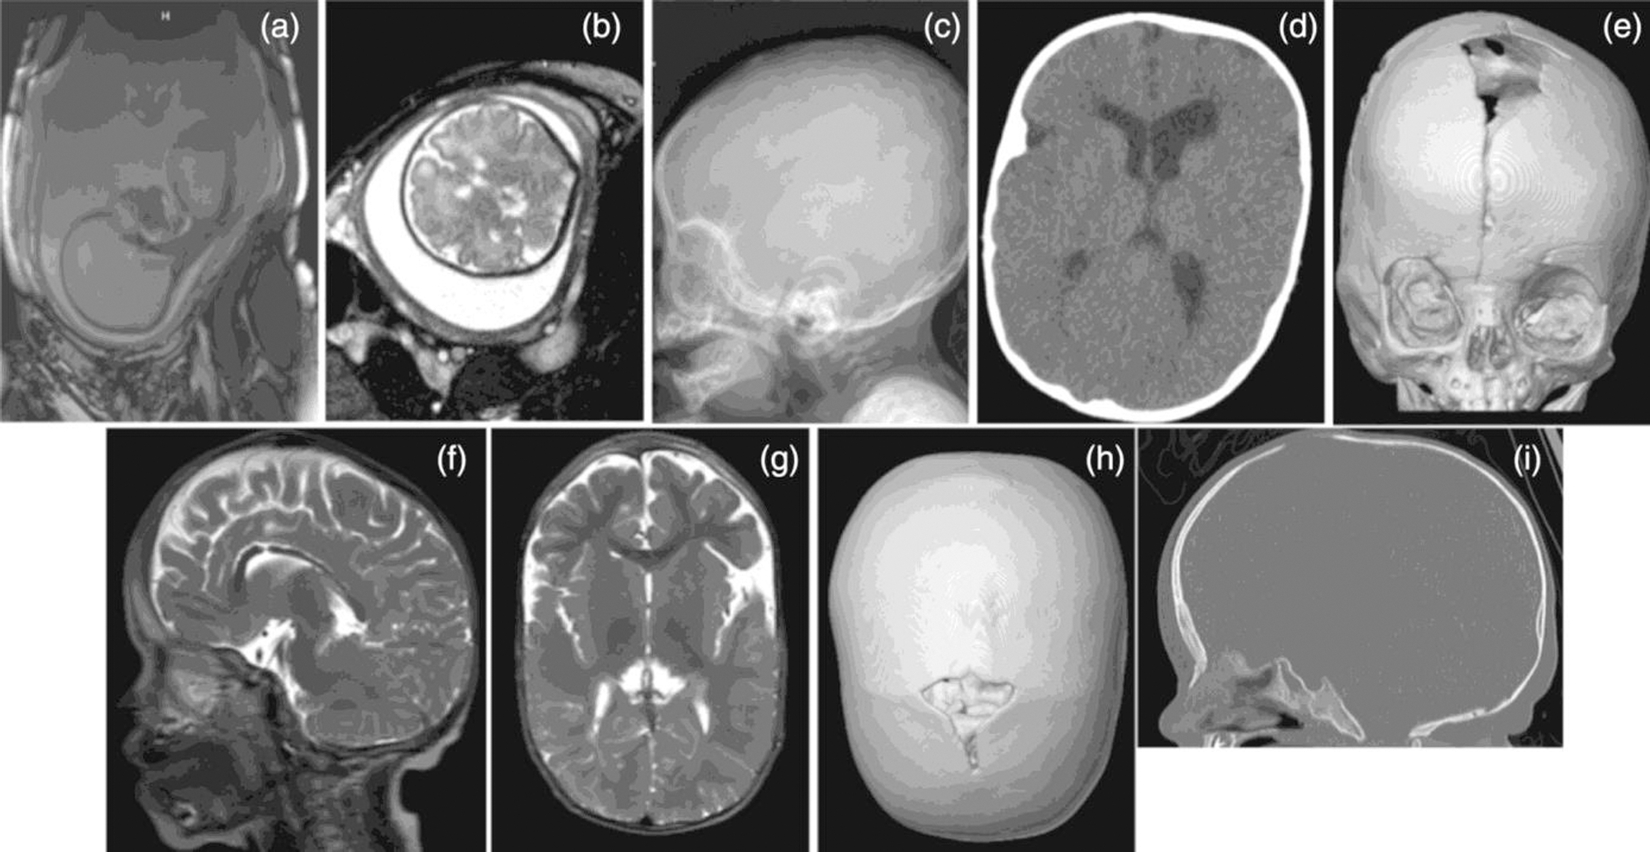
Imaging demonstrating craniosynostosis in Individuals 1–5. (a, b, Individual 1) (a) sagittal view on prenatal MRI; (b) transverse view on prenatal MRI. (c, Individual 2) (c) sagittal view on x-ray. (d, e, Individual 3) (d) transverse view on CT; (e) coronal view of a model of skull. (f, g, Individual 4) (f) sagittal view on MRI; (g) transverse view on MRI. (h, i, Individual 5) (h) CT scan with 3D reconstruction with near complete fusion of sagittal suture, premature closure of lambdoid, coronal, and squamosal sutures; frontal bossing; anterior fontanelle remains patent; (i) frontal bossing, j-shaped sella.
